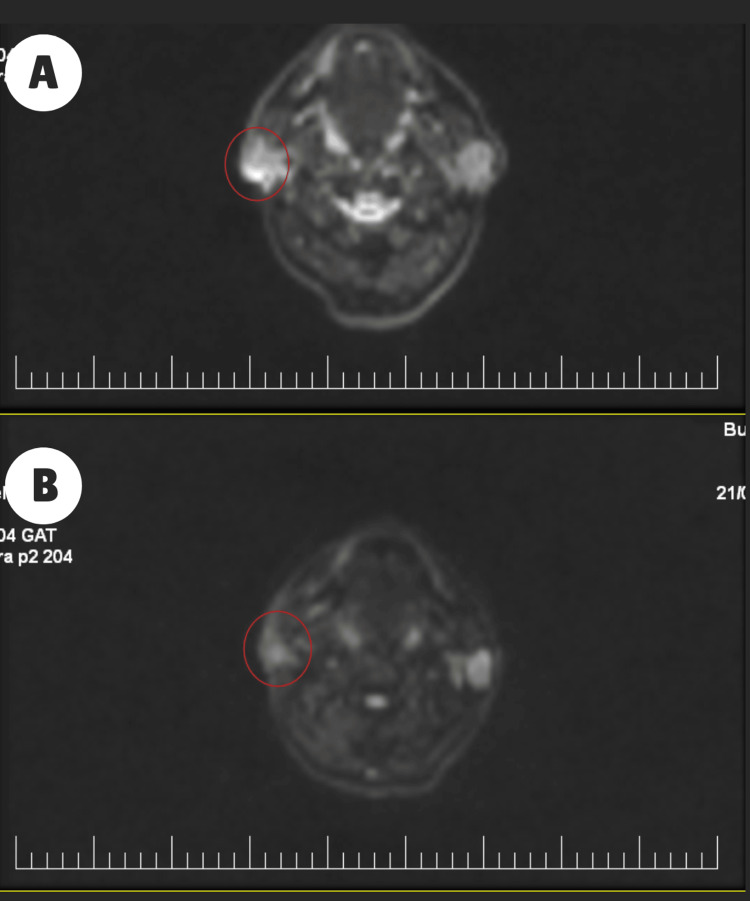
Diffusion-MRI showing the evolution of the parotid mass (red circle) before RT (A) and after RT (B). MRI: magnetic resonance imaging; RT: radiotherapy
